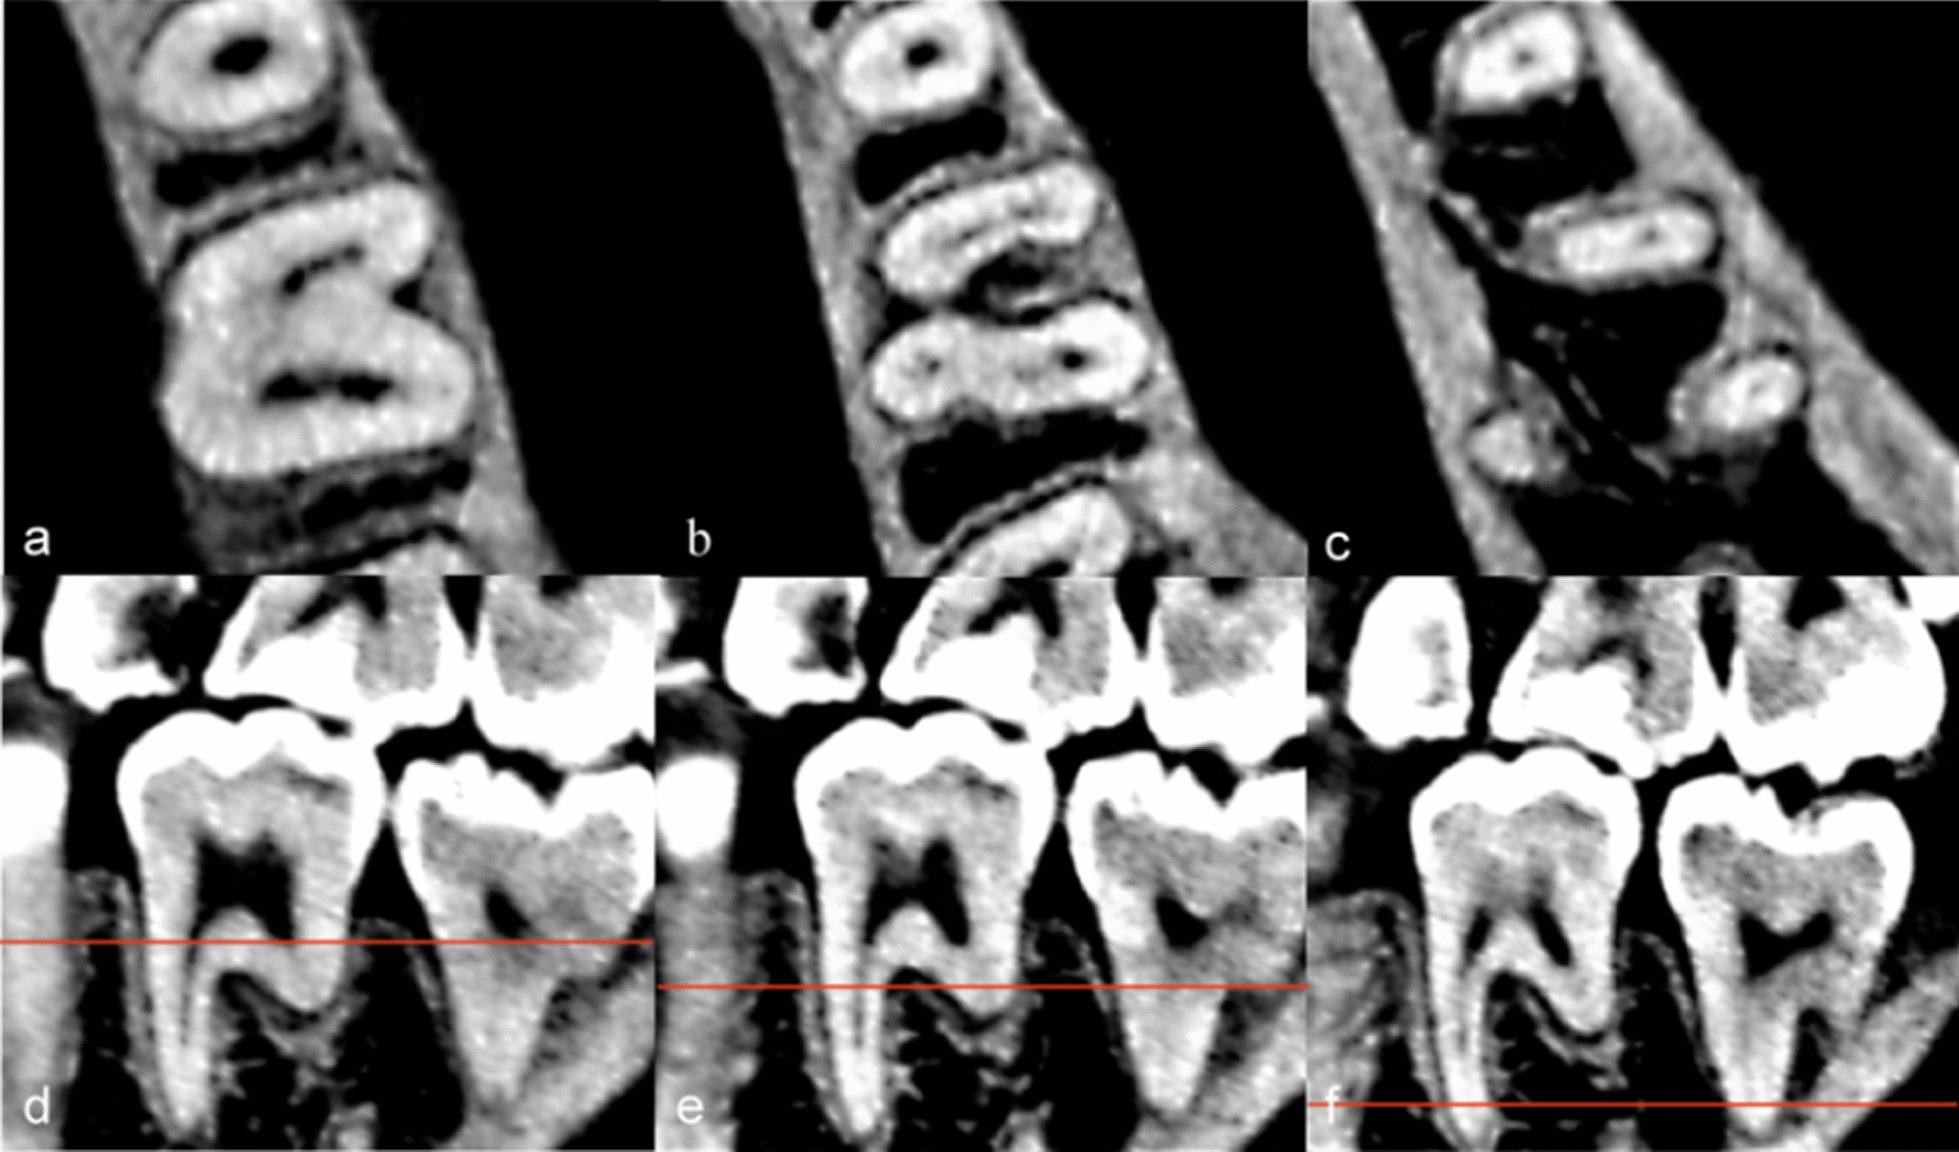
CBCT images of the 3-2-1 root canal system type. a–c were CT images with different cross sections: a Three canals can be seen at upper 1/3 of root canal; b There were two canals at middle1/3 of root canal; c two root canals eventually fused into one apical foramen; d–f are sagittal images
